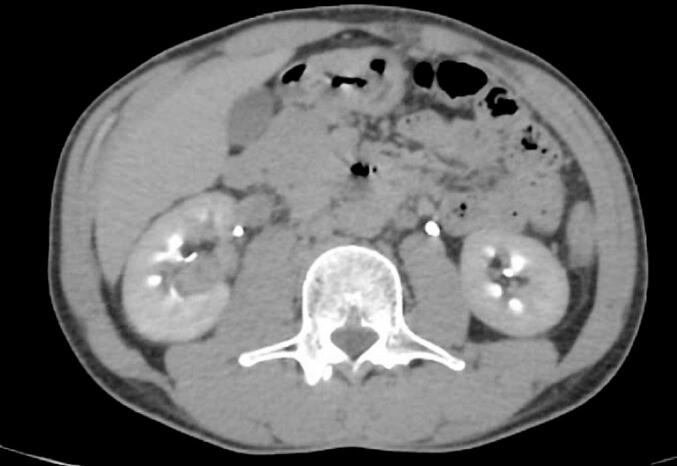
CT revealed a tissue mass measuring 30 × 14 mm in the right renal pelvis.
